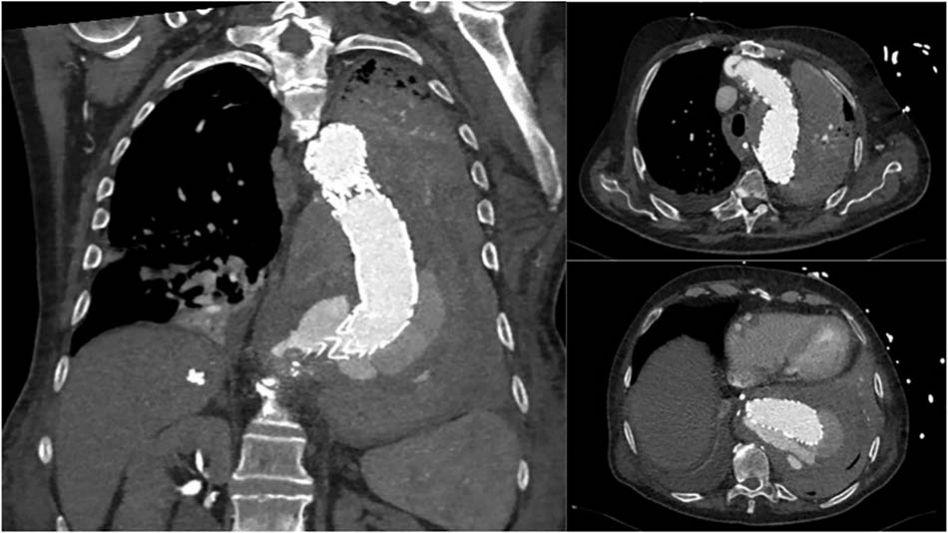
Coronal and axial CT thoracic aortogram indicating large Type 1 endoleak with large left haemothorax.
